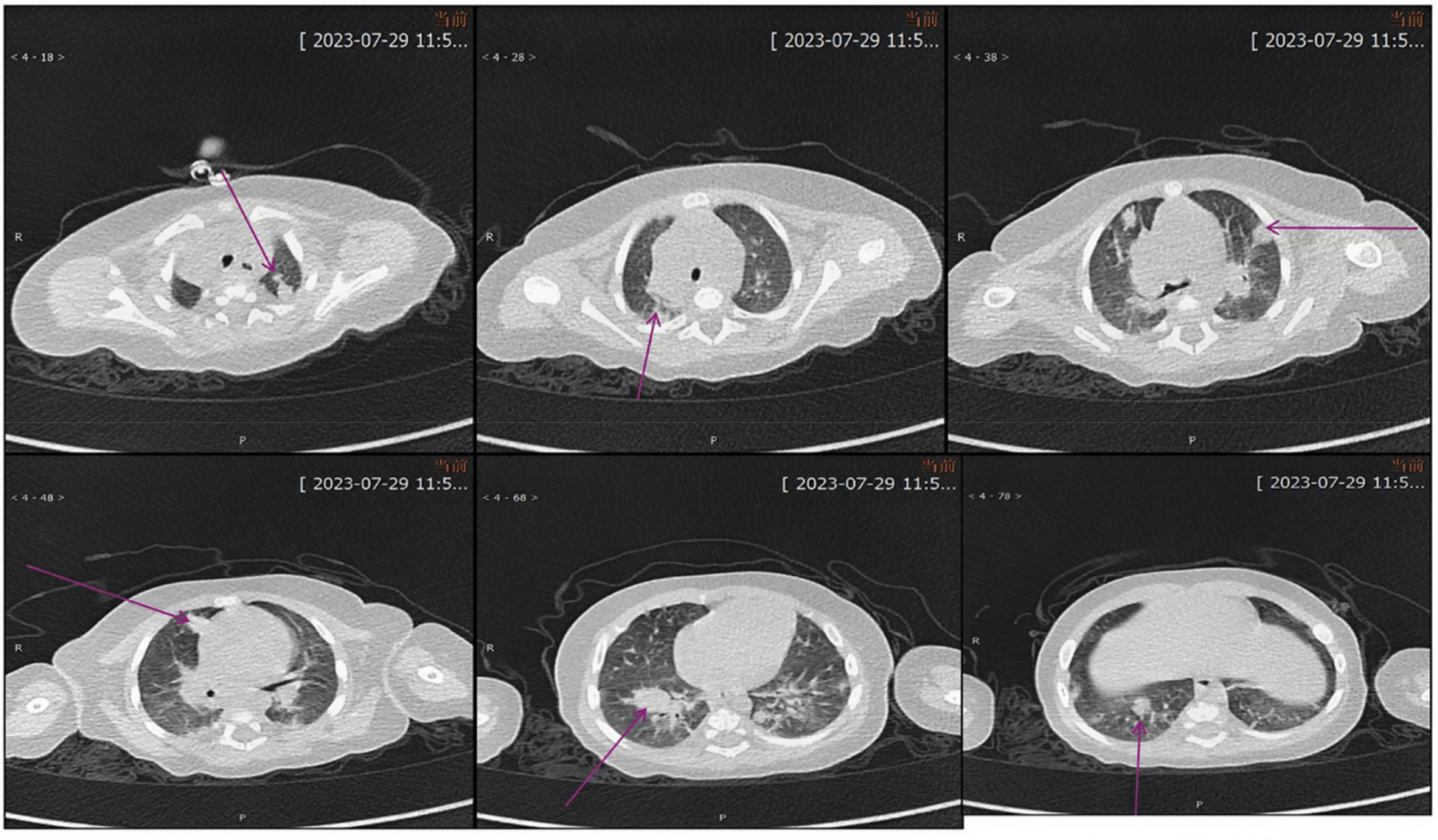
Contrast-enhanced chest CT performed on July 29, 2023 demonstrated multiple pulmonary lesions accompanied by mediastinal lymphadenopathy.
